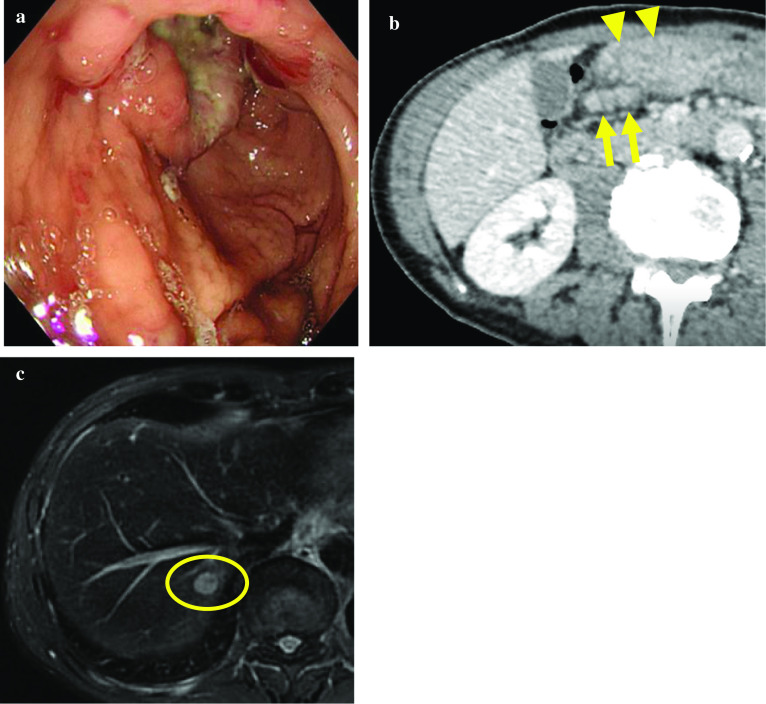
Image findings at the initial hospital visit (before any treatment). a Upper gastrointestinal endoscopy showed a type 2 circumferential tumor with a gastric outlet obstruction located at the gastric antrum. b Abdominal contrast-enhanced CT revealed a thickened gastric wall at the pre-pyloric area (triangle) and two swollen regional lymph nodes at station No. 6 (arrow). c On MRI, a highly enhanced lesion measuring 10 mm in diameter was detected in the liver S7 (circle)
